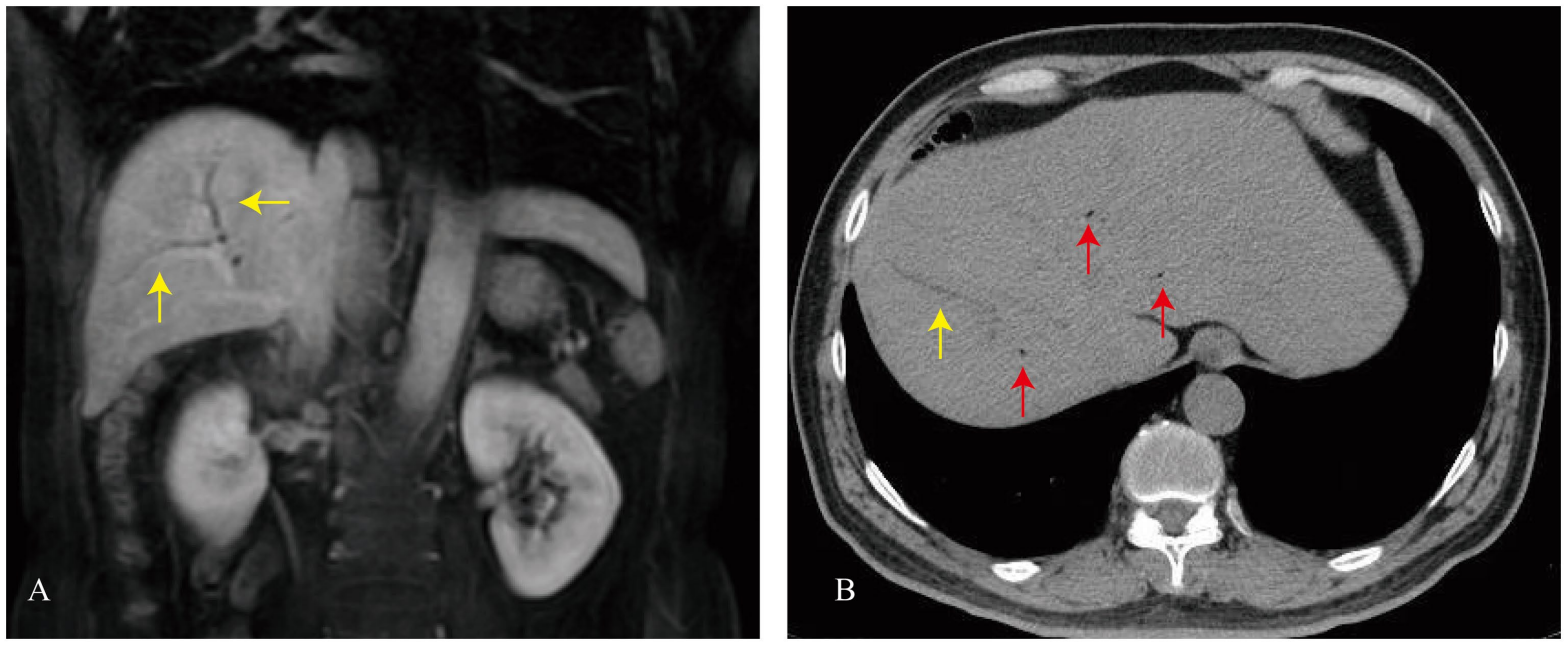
(A) MRI of the abdomen showing mild intrahepatic bile duct dilation (yellow arrow). (B) CT of the abdomen showing pneumobilia (red arrow) and mild intrahepatic bile duct dilation (yellow arrow).
